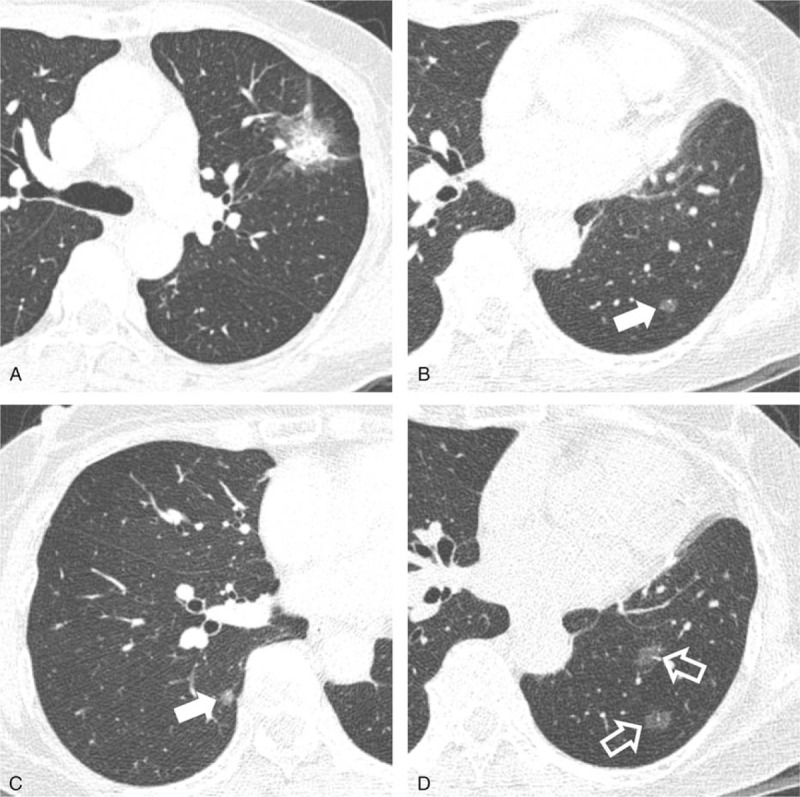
A 69-year-old female patient who underwent left upper lobectomy for a part-solid GGN-type adenocarcinoma. A, Preoperative chest CT shows a part-solid GGN in the right upper lobe of the lung. The whole nodule including GGO parts and its solid part were measured as 3.0 and 1.8 cm, respectively. Visceral pleural invasion and lymphatic invasion were observed on pathologic examination. B, C, Follow-up chest CT performed 2 years after surgery shows multiple pure GGNs in the bilateral lungs (arrows). D, Subsequent follow-up chest CT performed 6 months later than (B) shows an increased size and number of multiple pure GGNs (open arrows), which were determined as metastases through multidisciplinary discussion.
